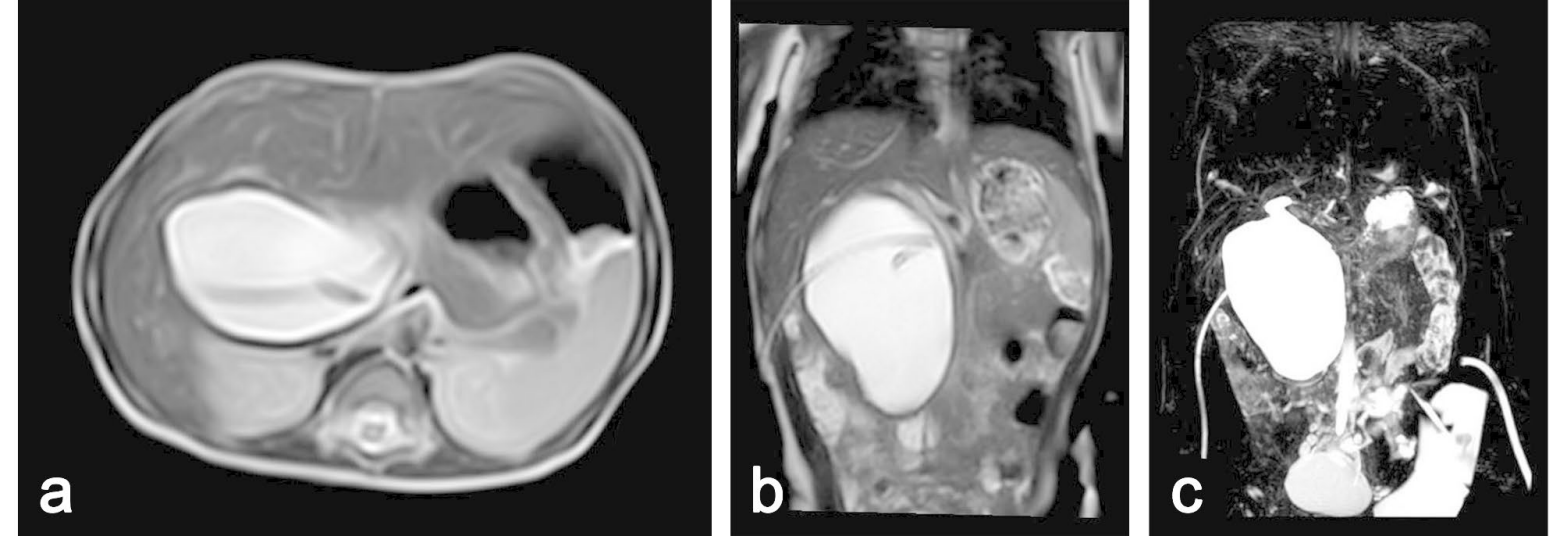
Images of biliary drain after US-guided PBD. (A) Axial T2-weighted MR image after US-guided PBD, Case 2. (B) Coronal T2-weighted MR image after US-guided PBD, Case 2. (C) MRCP image after US-guided PBD, Case 2
